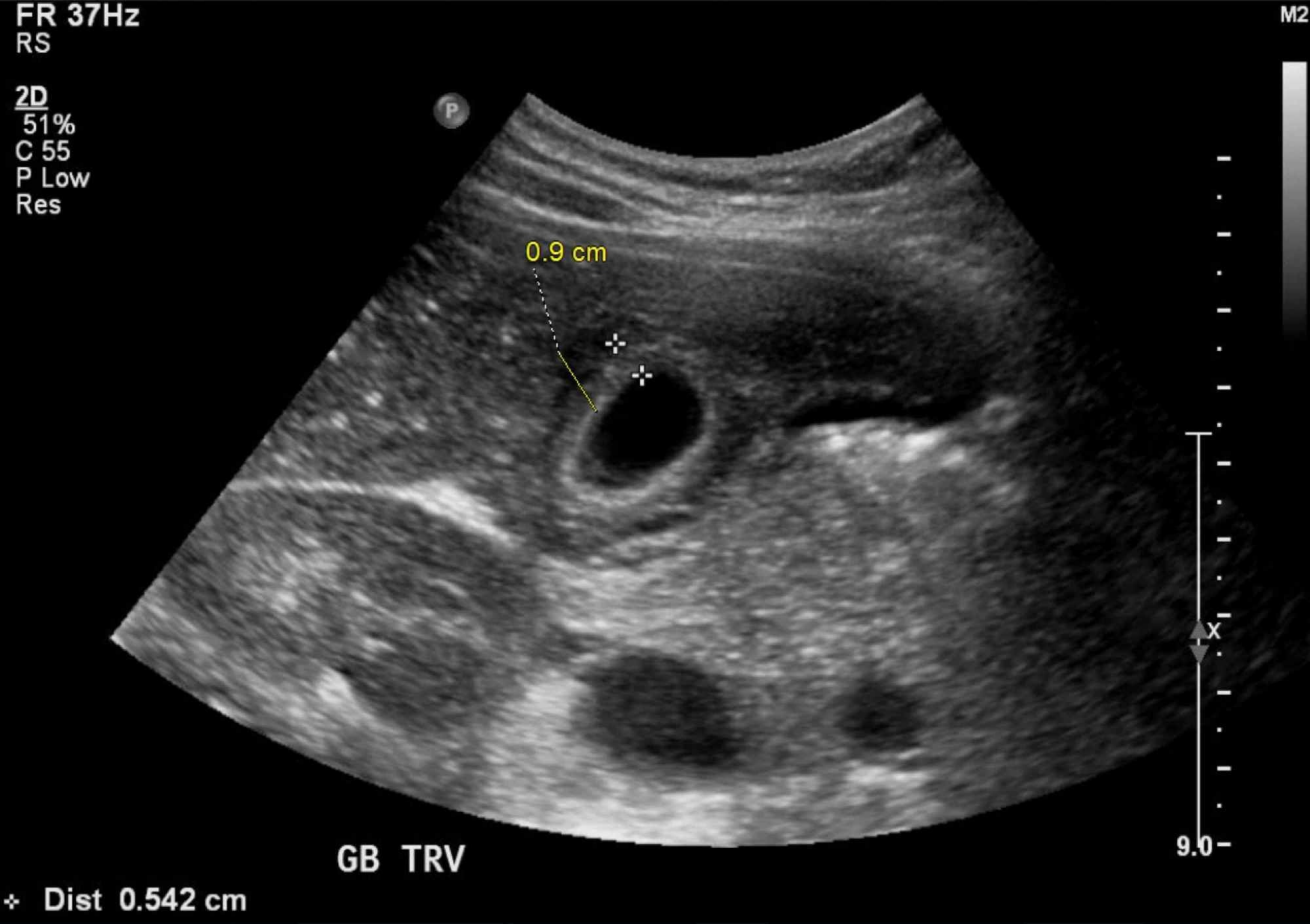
Abdominal ultrasound Abdominal ultrasound showing gallbladder dilatation more than 3 mm and pericholecystic fluid
